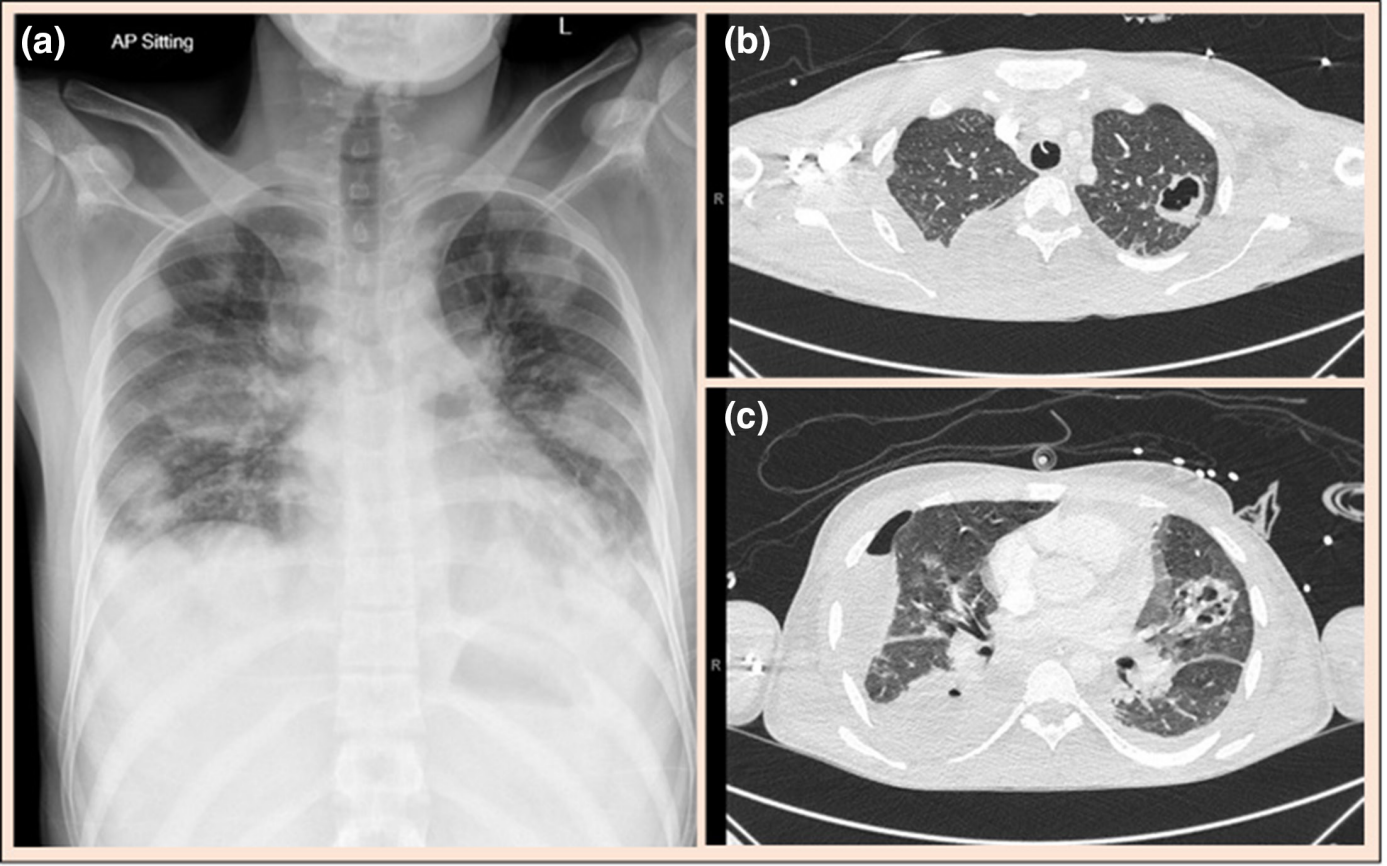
Chest X-ray (a) and axial CT slices (b and c) demonstrating multiple cavitary lesions present in both lungs.
